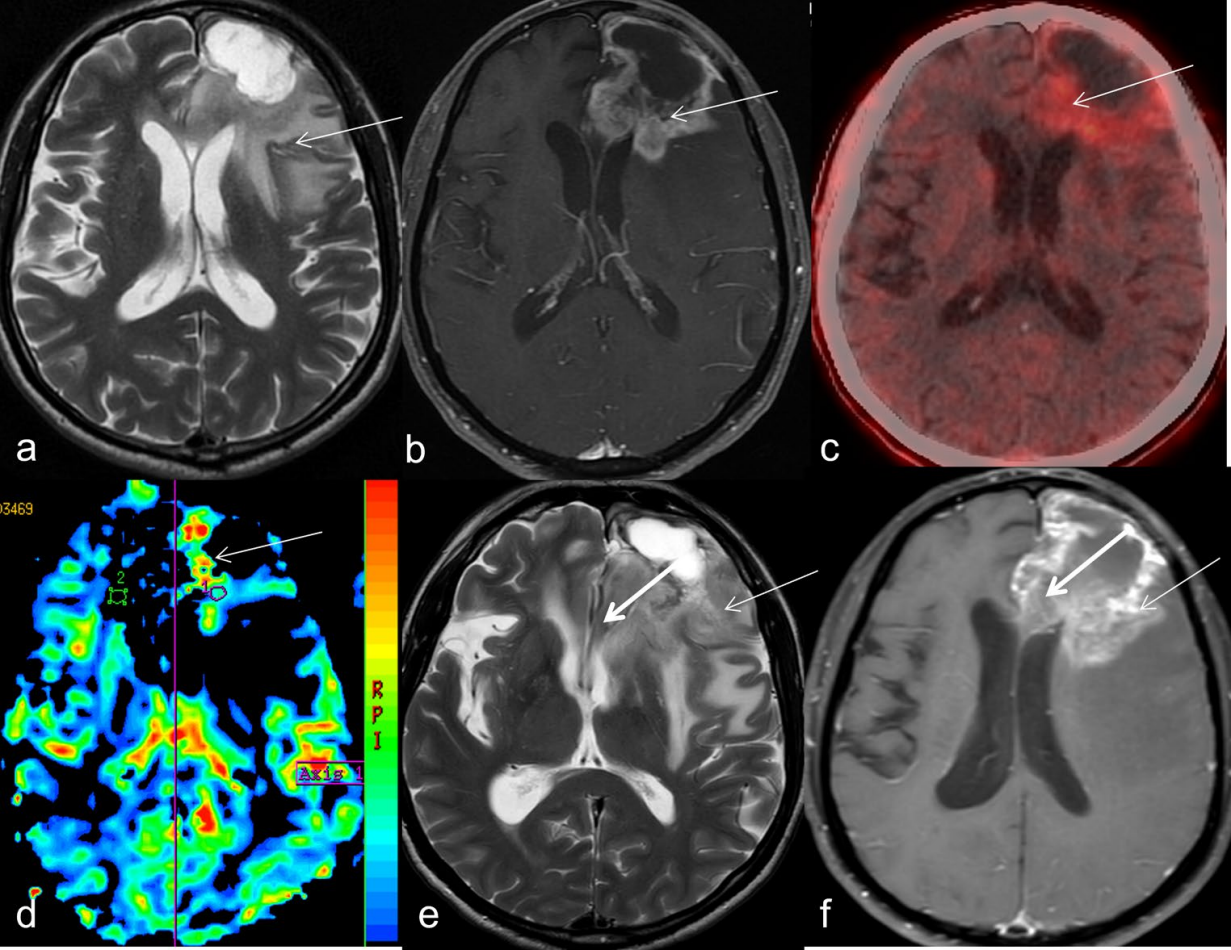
Disease recurrence on FET PET. 52-year-old male, case of anaplastic astrocytoma, post excision, received adjuvant radiotherapy along with temozolomide (TMZ), followed by 12# maintenance TMZ, had a disease-free interval of 14 months. T2-weighted axial image (A-arrow) on follow-up MRI showed iso-to-hypointense areas in left frontal region, seen as swiss-cheese pattern of enhancement on axial post-contrast T1-weighted image (B-arrow). Patient underwent FET PET for characterisation of lesion, which showed high T-Wm ratio of 3.6 suggestive of disease recurrence. Focal areas of hyperperfusion were seen (D-arrow). Since patient was well-preserved clinically, he was followed up after 3 months with MRI, which showed increase in extent of infiltration on T2-weighted (E- arrow) and post-contrast-T1 weighted image (F- arrow). MDC discussions corroborated the FET and follow up MR findings and concluded that patient had unequivocal disease recurrence and was started on salvage treatment
